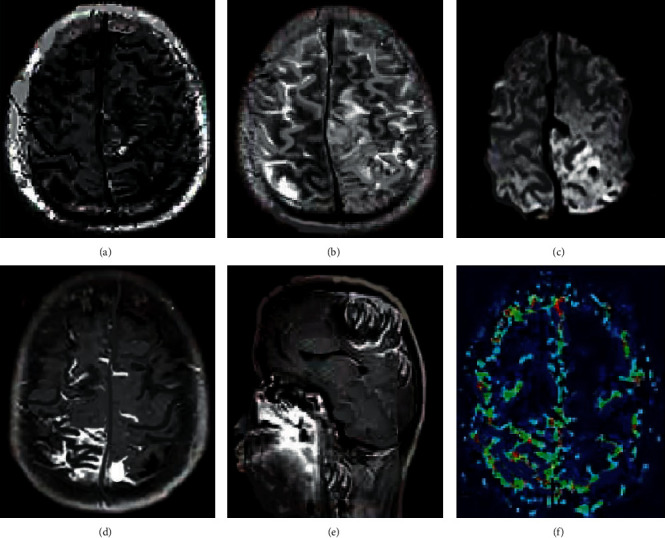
MRI images of single intracranial metastatic melanoma.
